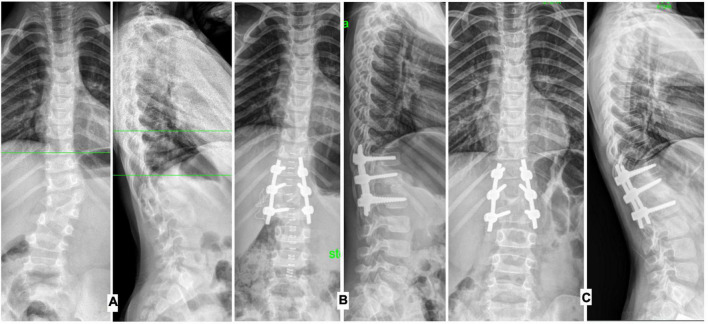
Radiographs of the spine in the frontal and lateral projections, operated at the age of 3 years 6 months: (A) before surgical treatment, (B) on the 6th day after surgical treatment, (C) 3 years after correction of congenital spinal deformity.
